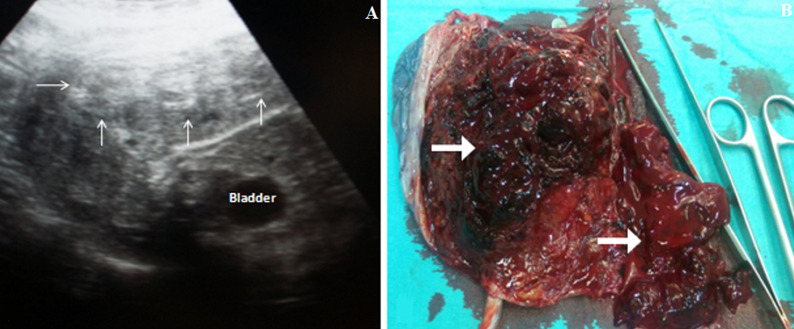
A) obstetrical ultrasound objectified the retroplacental hematomas (white arrows); B) macroscopic view of the adherent blood clot on maternal surface (white arrows)
